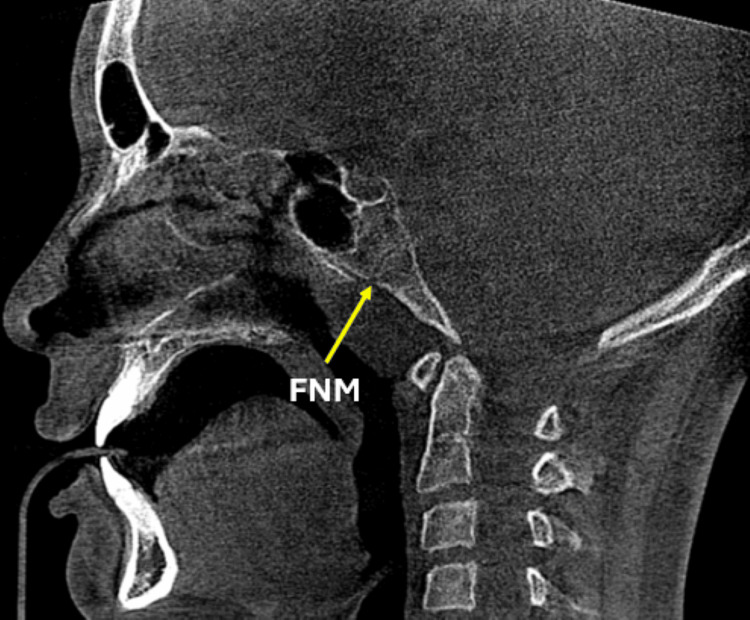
Sagittal CBCT section showing Fossa Navicularis Magna (FNM) as a well-corticated notch-like defect on the inferior aspect of the clivus. CBCT, cone-beam computed tomography.
